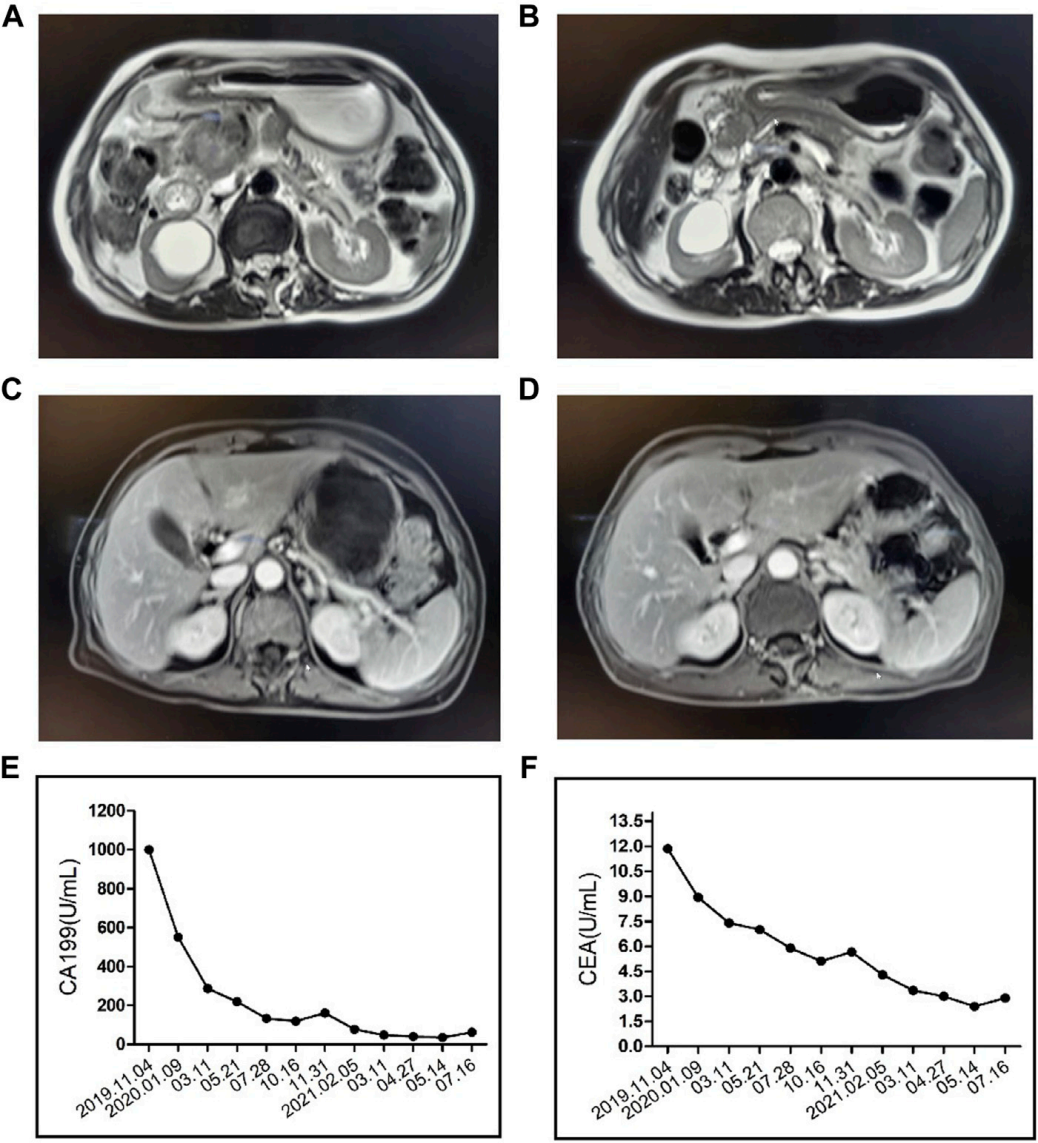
Contrast-enhanced Magnetic Resonance Imaging (MRI) of the upper abdomen showed the patient’s pancreatic carcinoma before (A) and after (B) the treatment of PD-1, HIFU and Gemcitabine. MRI showed the patient’s liver metastasis before (C) and after (D) the treatment. CA199 (E) and carcinoembryonic antigen (CEA) (F) index of the patient.
